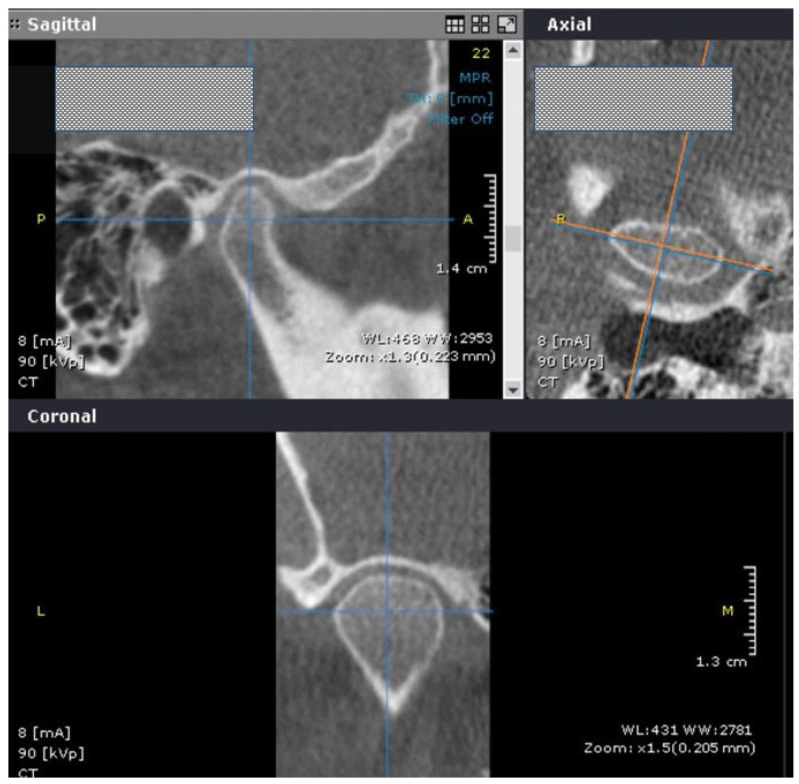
Cone beam computer tomography (CBCT) image in the sagittal, axial, and coronal views showing the slices that were selected for the measurements.
